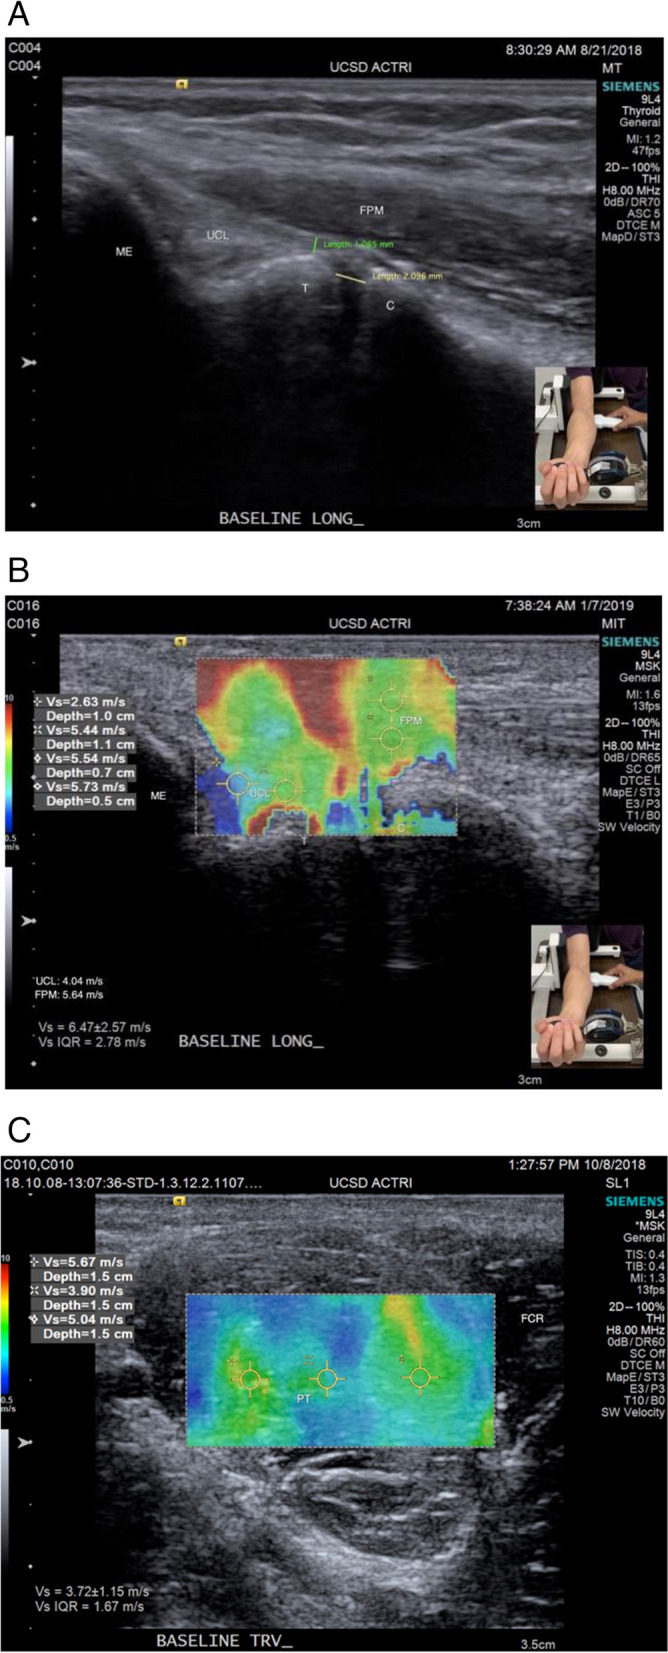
a: Baseline longitudinal ulnar collateral ligament (UCL) ultrasound image with reference measurements for UCL thickness and ulnohumeral joint space width (JSW). Ultrasound probe positioning as shown in Fig. 1. b: Baseline longitudinal UCL shear wave elastography image with demonstration of sampling method of shear wave velocity for UCL and for the flexor pronator mass. Longitudinal measurements comprised the region of flexor pronator mass muscle overlying the UCL. Ultrasound probe positioning as shown in Fig. 1. c: Baseline transverse pronator teres (PT) elastography image with demonstration of sampling method of muscle shear wave velocity. Measurements were taken in the transverse PT muscle body. Acuson SC3000 with a velocity range set at 0.5 to 10 m/s. Ultrasound robe positioning is orthogonal to that shown in Fig. 1
